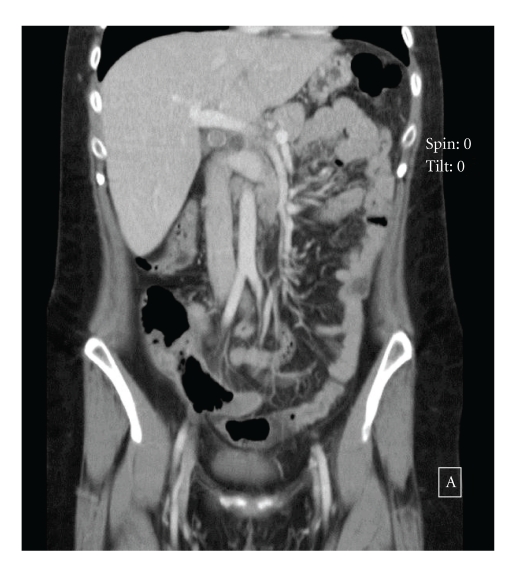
CT scan (coronal view) of the abdomen and pelvis showing an appendiceal mucocele with intraluminal gas and wall edema.
